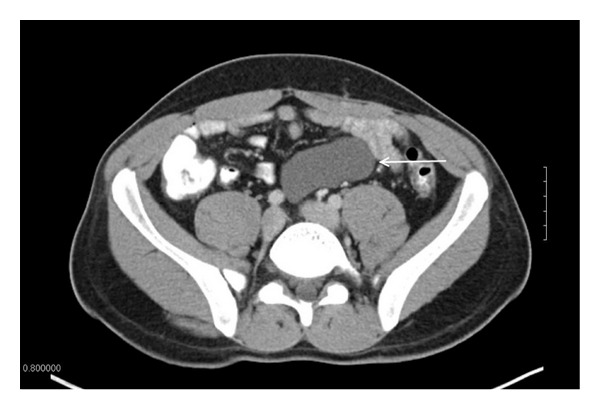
CT of the abdomen and pelvis shows an 8 cm homogenous cystic lesion (white arrow).
